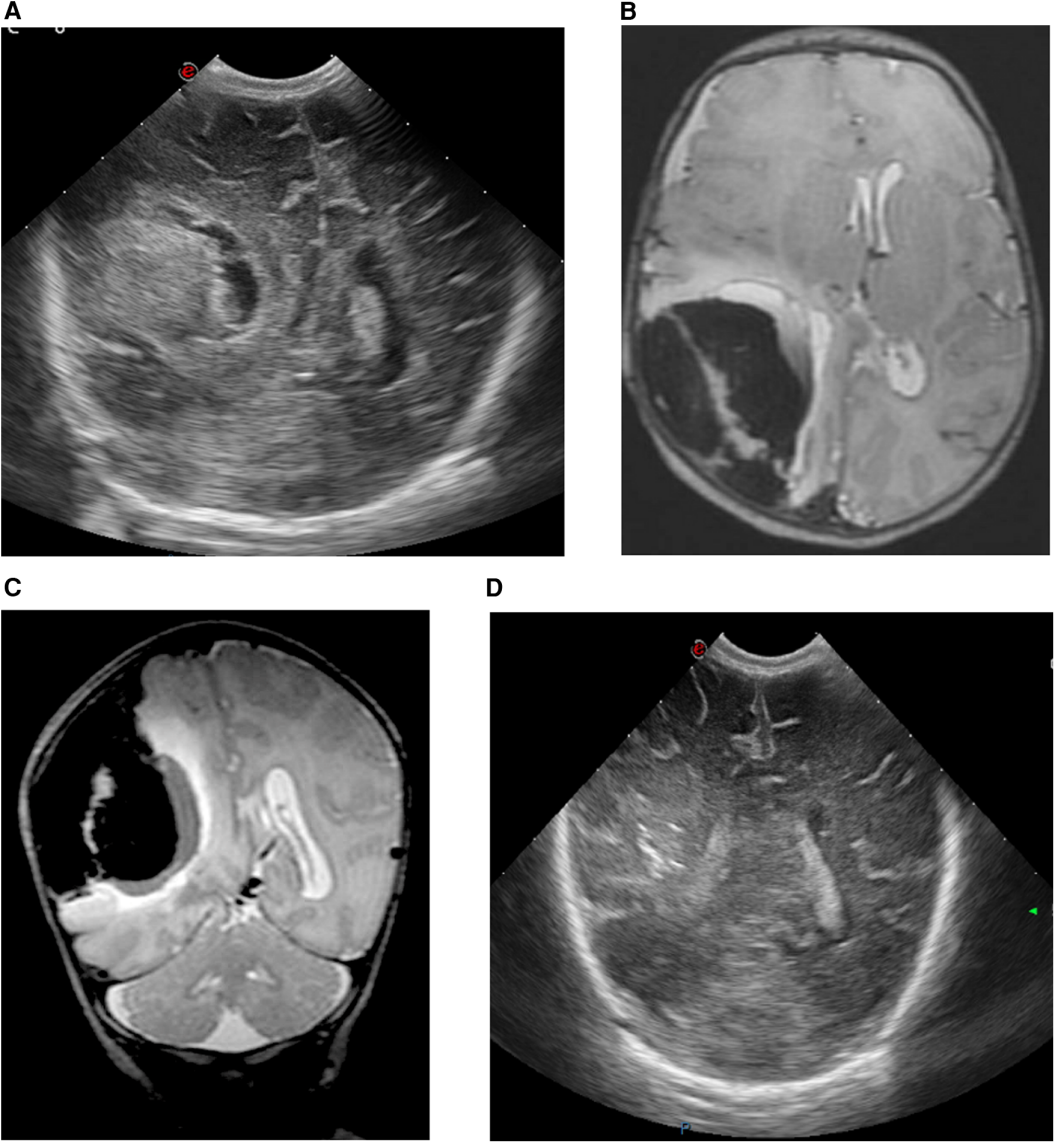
cUS and MRI brain findings of case 2. (A) cUS coronal view, showing a large echogenic parieto-occipital lesion at the right side, presumably extra-axial hemorrhage with midline shift to the left side. (B) MRI T2-weighted sequence, axial view, and (C) MRI T2-weighted sequence, coronal view, showing a large extra-axial hemorrhage in the parieto-occipito-temporal region on the right side with a maximal thickness of 43 mm and a midline shift of 8 mm to the left. (D) cUS coronal view, after craniotomy showing a decrease in the size of the hematoma, reduction of the midline shift and slender ventricles.
